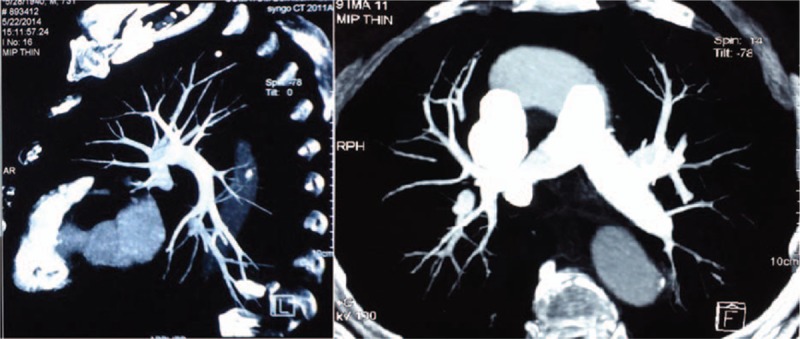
CTPA showed that most of the PTE in the left superior and left inferior pulmonary arteries was dissolved in case 2. CTPA = computed tomographic pulmonary angiography, PTE = pulmonary thromboembolism.
